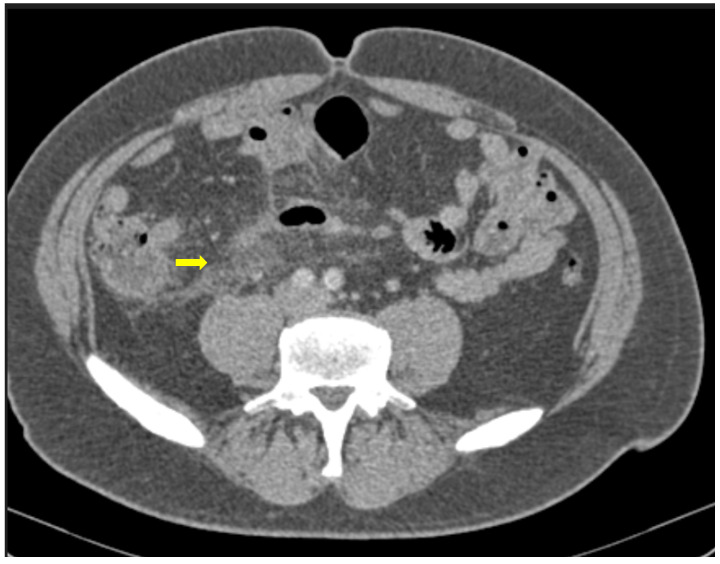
55-year-old male patient with COVID-19 pneumonia with right lower quadrant pain. CT on admission demonstrating a thickened appendix and proximal fat stranding (yellow arrow), indicating acute appendicitis.
